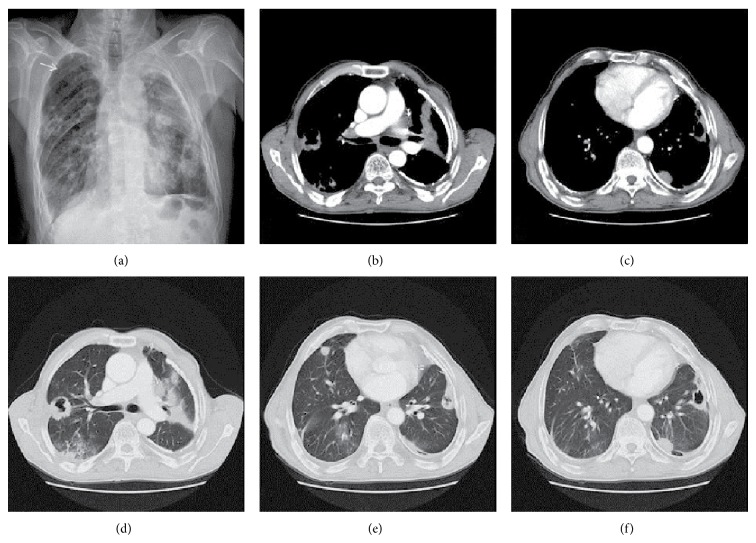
(a) X-ray imaging of lung. Bifid costa, multiple irregular cavity, and metastatic nodules at the lungs. (b)–(f) CT imaging of thorax: (b) irregular cavitary and nodular metastatic lesions at right upper pulmonary segments and pleural calcifications. (c) Left pulmonary irregular cavitary and nodular metastatic lesions. (d), (e), and (f) Multiple solid and cavitary metastatic pulmonary nodules.
